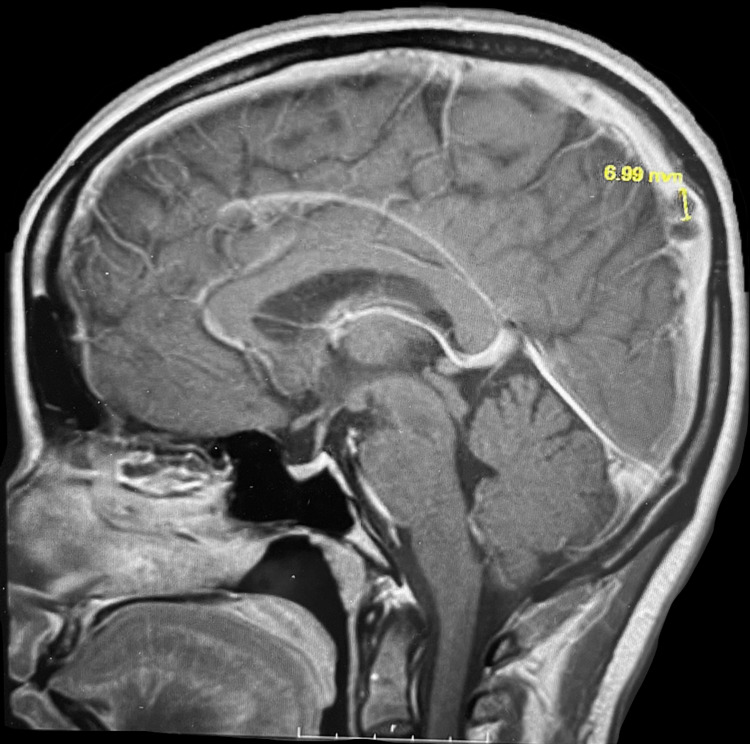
MRI of Brain With a Sagittal Sinus Filling Defect
